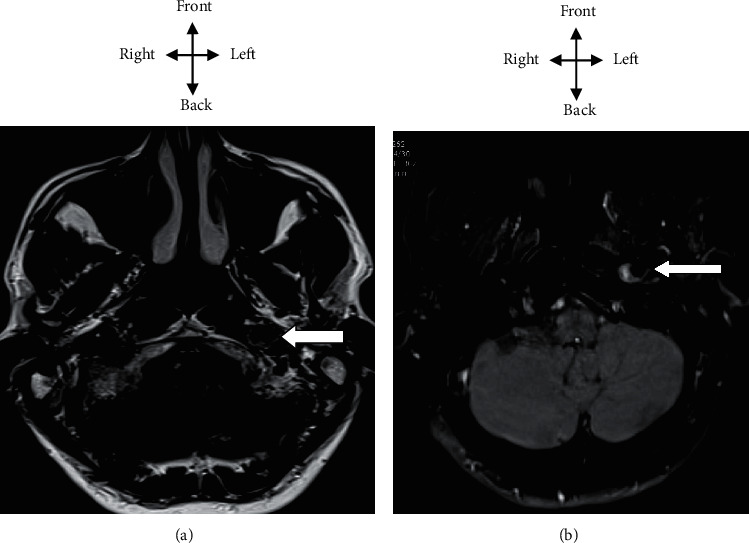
MRI of the neck vessels, T2 FLAIR axial (a) and T1 FAT-SAT axial (b), hyperintense subintimal hematoma.
